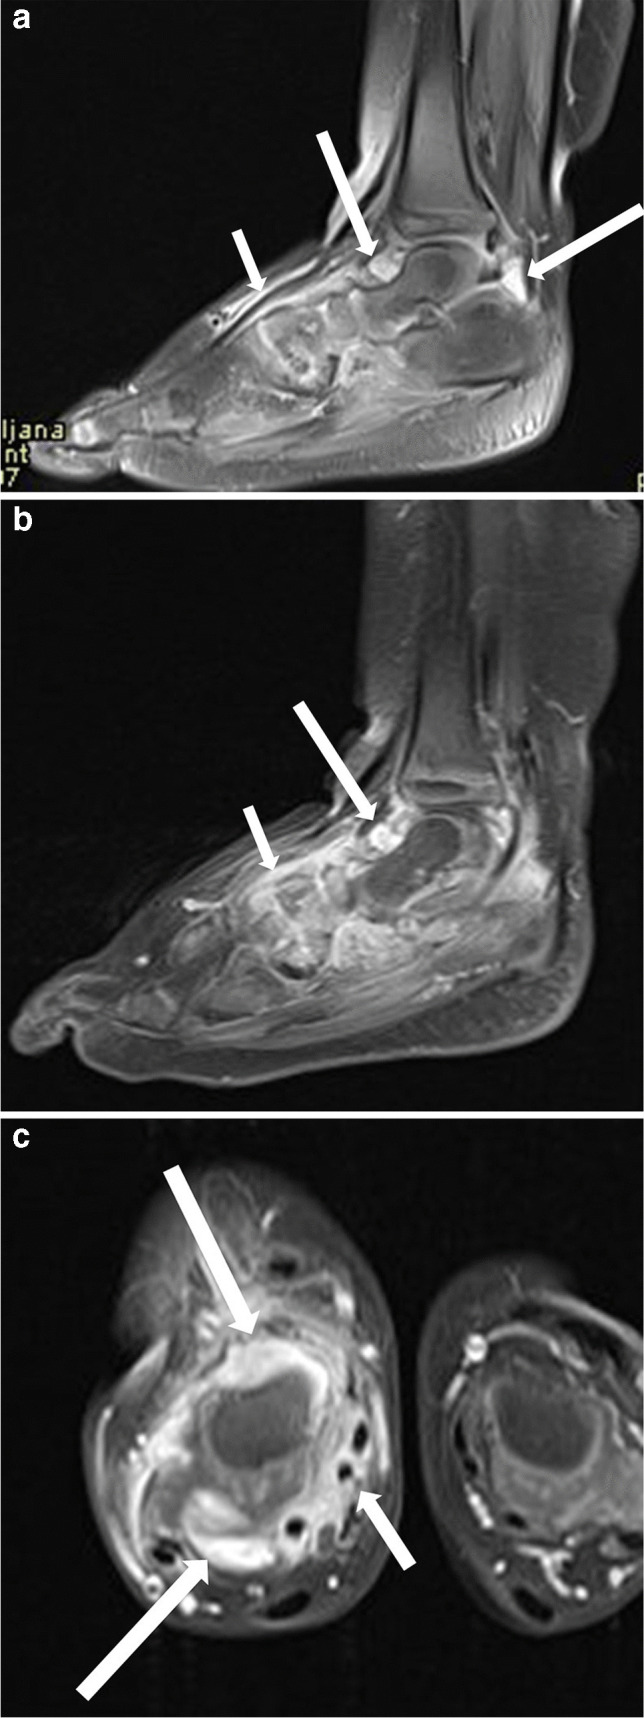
Magnetic resonance imaging of the right ankle in a 2-year-old girl with juvenile idiopathic arthritis (HLA B27 negative, anti-nuclear antibodies positive, same patient as in Fig. 5). a Sagittal proton-density-weighted fat suppressed image shows marked synovial proliferation and effusion of the anterior and posterior recesses of the ankle joint (*long arrows*) and around the extensor tendons (*short arrow*)—signs of synovitis and tenosynovitis. b Sagittal postcontrast T1-weighted fat-suppressed sequence shows marked synovial proliferation and enhancement of the anterior recess of the ankle joint (*long arrow*) and around the tarsal bones (*short arrow*)—signs of active synovitis. c Axial postcontrast T1-weighted fat-suppressed sequence shows marked synovial proliferation and enhancement of the anterior and posterior recesses of the ankle joint (*long arrows*) and around the flexor tendons (*short arrow*)—signs of active synovitis and tenosynovitis
